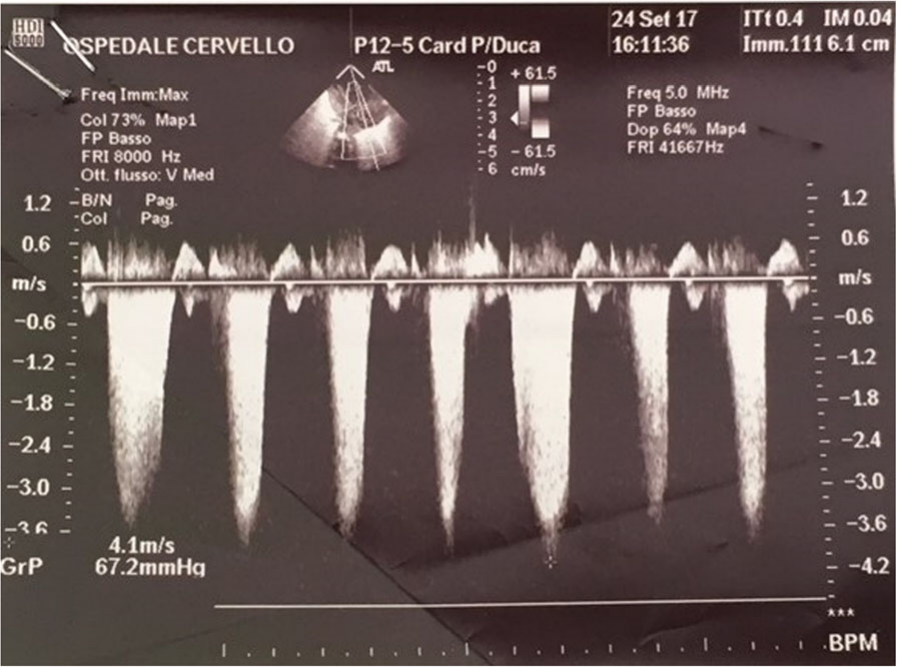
Doppler ultrasound showing severe pulmonary hypertension: velocity of tricuspid regurgitant jet up to 4.1 m/sec
